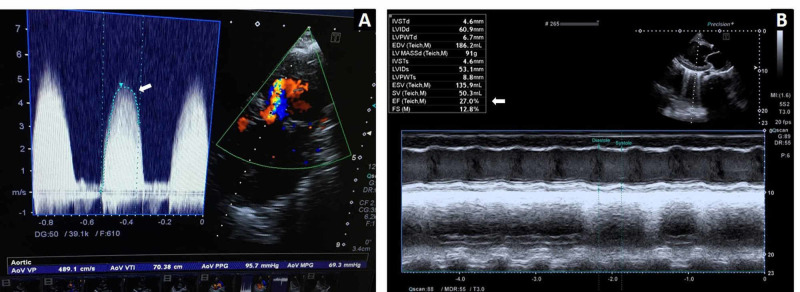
Color Doppler echocardiography by continuous-wave tracing in suprasternal view showing pressure gradient across aortic valve (A) and parasternal long-axis view showing left ventricular dimension and ejection fraction (B)
